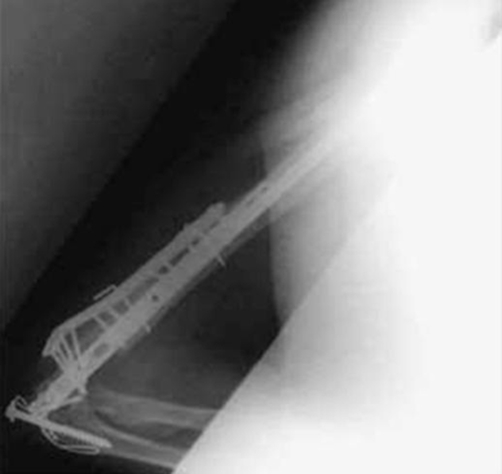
Lateral radiograph of left elbow post fixation with elbow in flexion.
